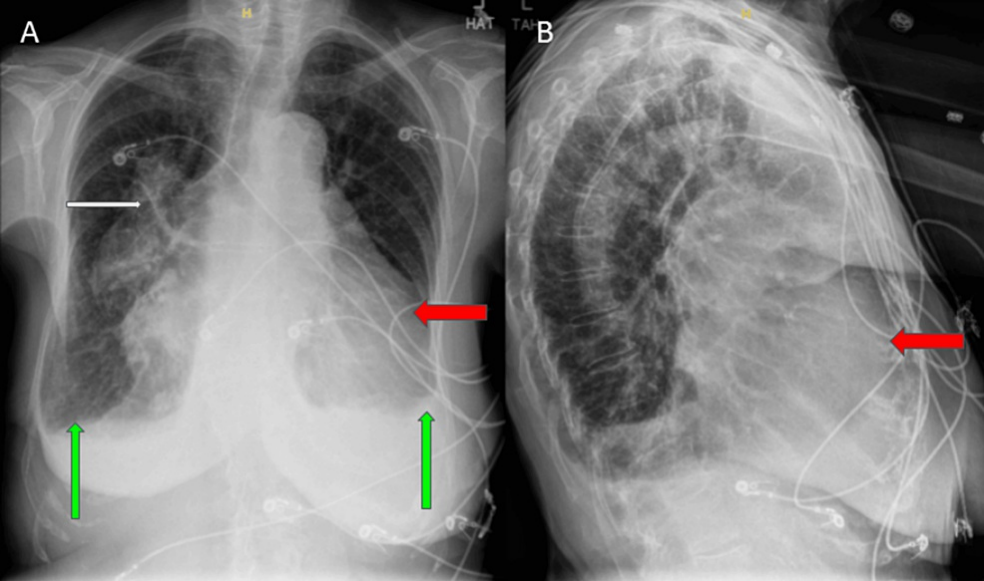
A: Chest X-ray showing globular cardiomegaly suggesting multi-chamber enlargement (indicated by the red arrow), interstitial pulmonary edema (indicated by the white arrow), and small bilateral pleural effusions (indicated by the green arrows). B: Chest X-ray showing cardiomegaly (indicated by the red arrow).
